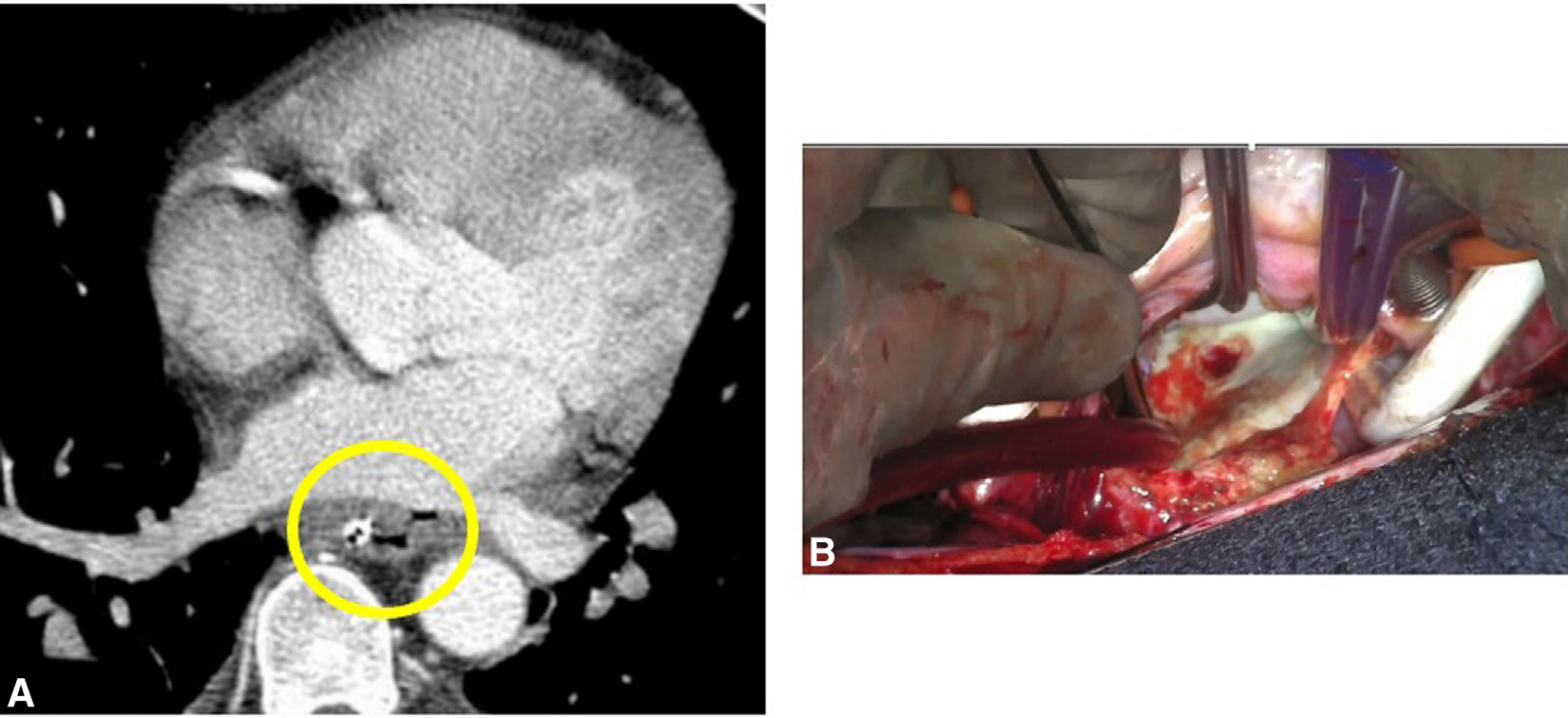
A, Computed tomography scan with air in mediastinum. B, Fistula tract from left atrium.
